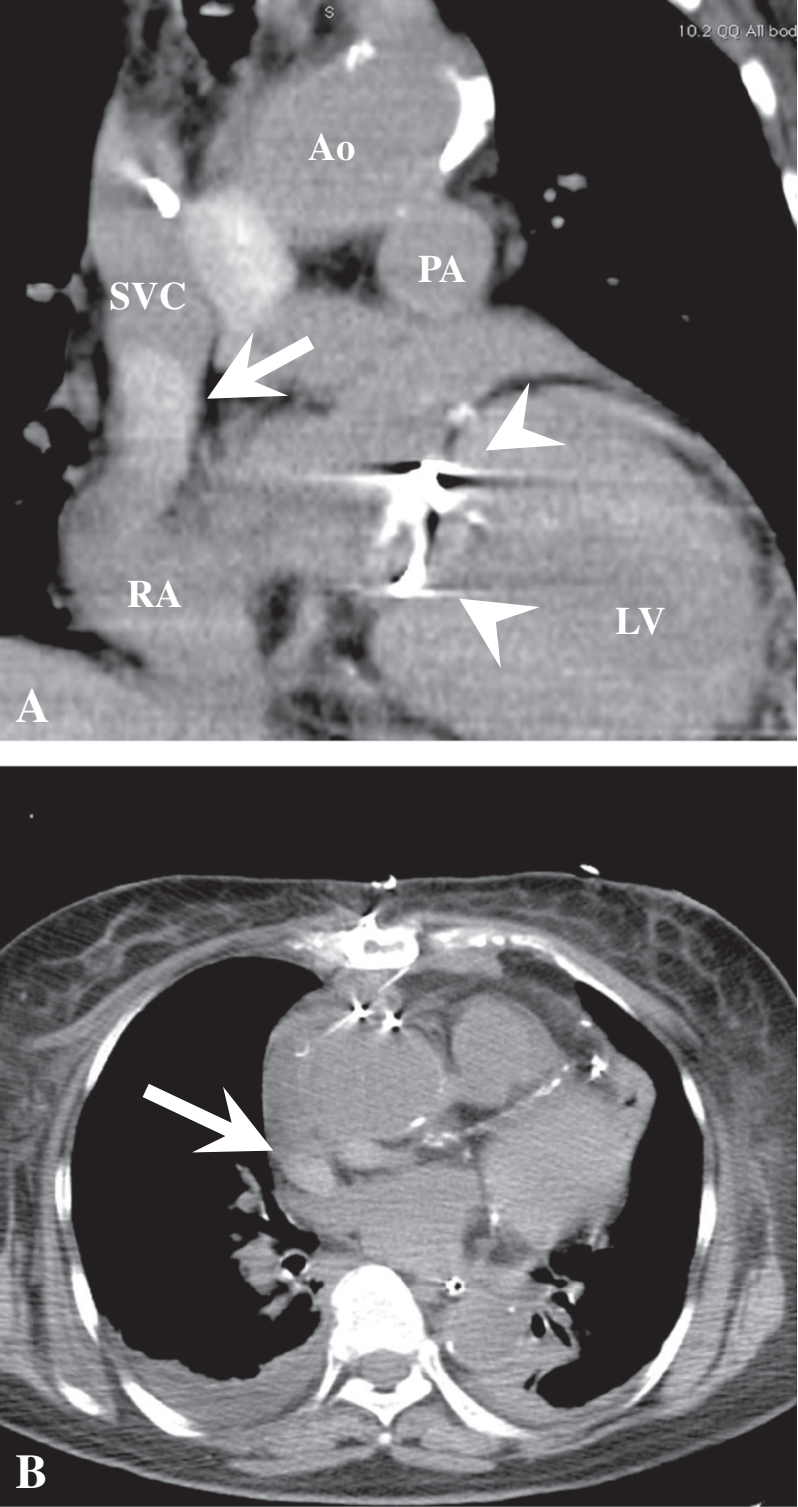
Planar computed tomography. A, Coronal view. B, Axial view. A high density area (arrow) confined at the terminal groove was observed. A mitral ring was observed (arrowhead). Ao: aorta, PA: pulmonary artery, LV: left ventricle, RA: right atrium, SVC: superior vena cava.
